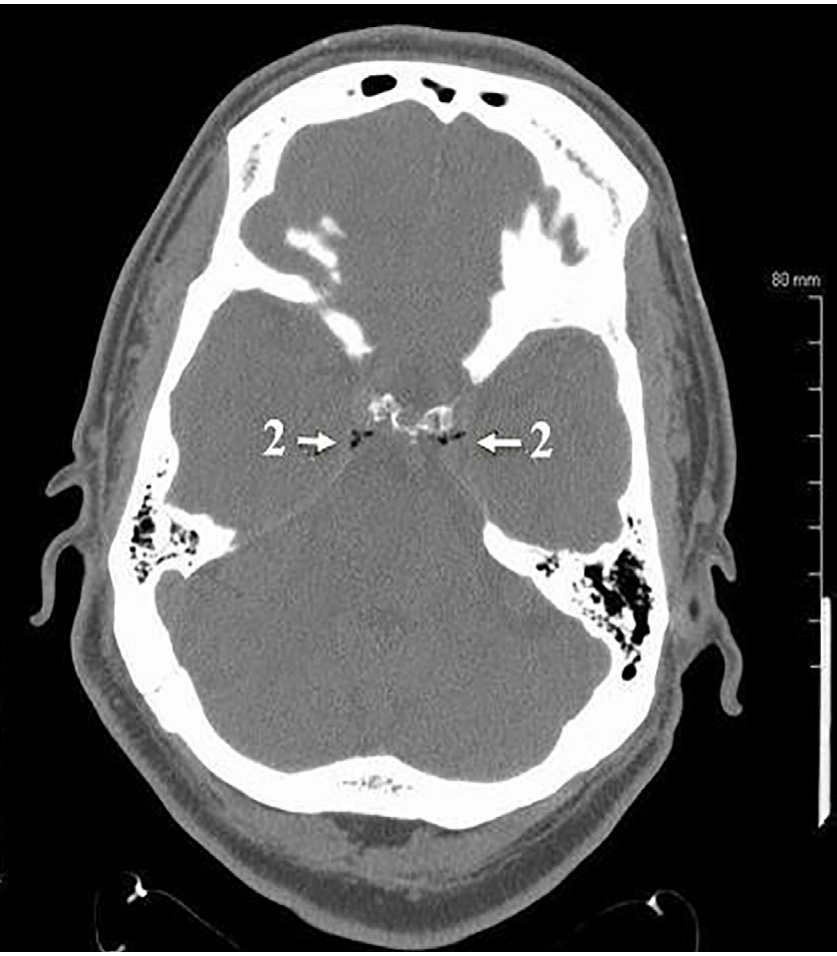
Intracranial air noted on patient’s computed tomography of the head. The arrows marked with 2 indicate multiple foci of gas within the cavernous sinus, part of the intracranial venous drainage system.
